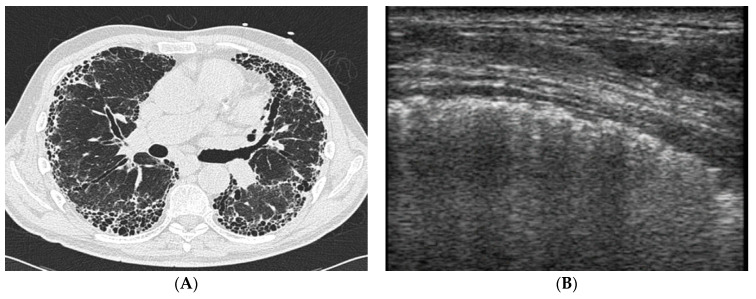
(A) HRCT scan of a patient with pSS showing bilateral subpleural honeycombing and traction bronchiectasis compatible with UIP pattern. (B) LUS performed on the same patient, with a 5–12 MHz linear probe showing marked irregularities of the pleural line.
